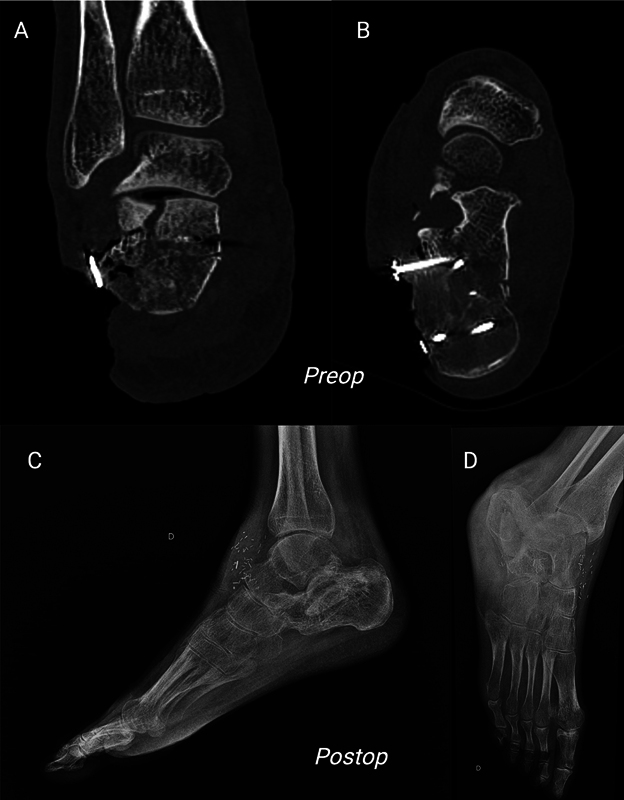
Imaging of the right ankle: Preoperative CT Scan Frontal
